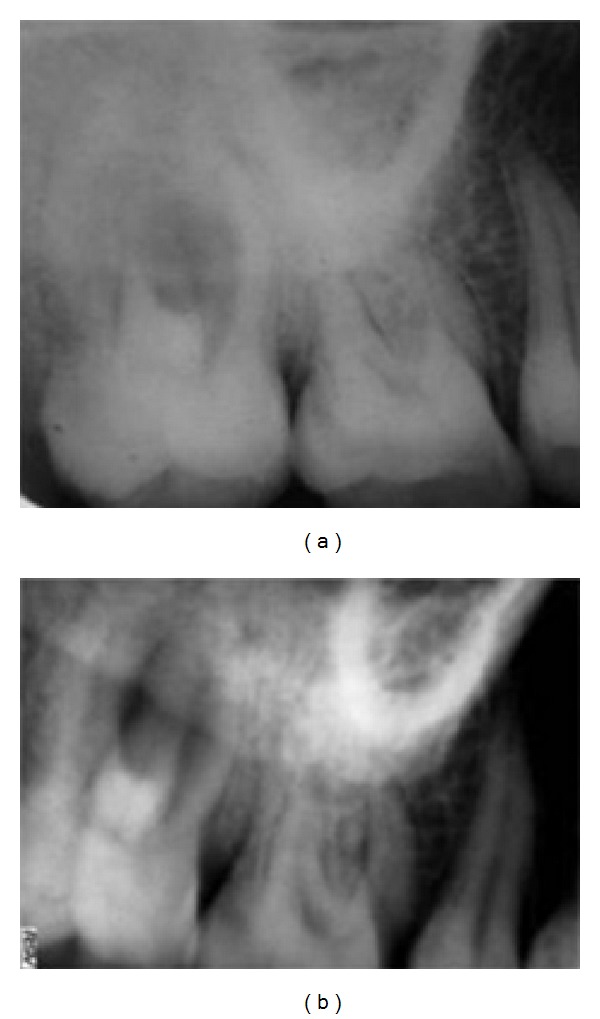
Preoperative radiograph with different angulations: complex root anatomy of maxillary right second molar.
